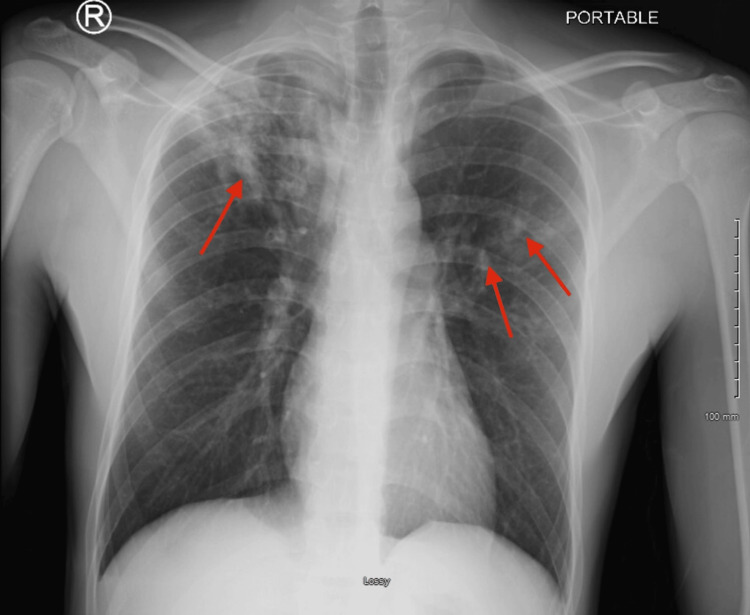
Chest radiograph demonstrating multifocal pulmonary opacities. A frontal chest radiograph demonstrates right upper-lobe airspace opacity and additional nodular opacities in the left upper and mid-lung fields (red arrows). These findings raised concern for multifocal infectious or granulomatous disease and prompted CT evaluation and airborne isolation. CT: computed tomography.
